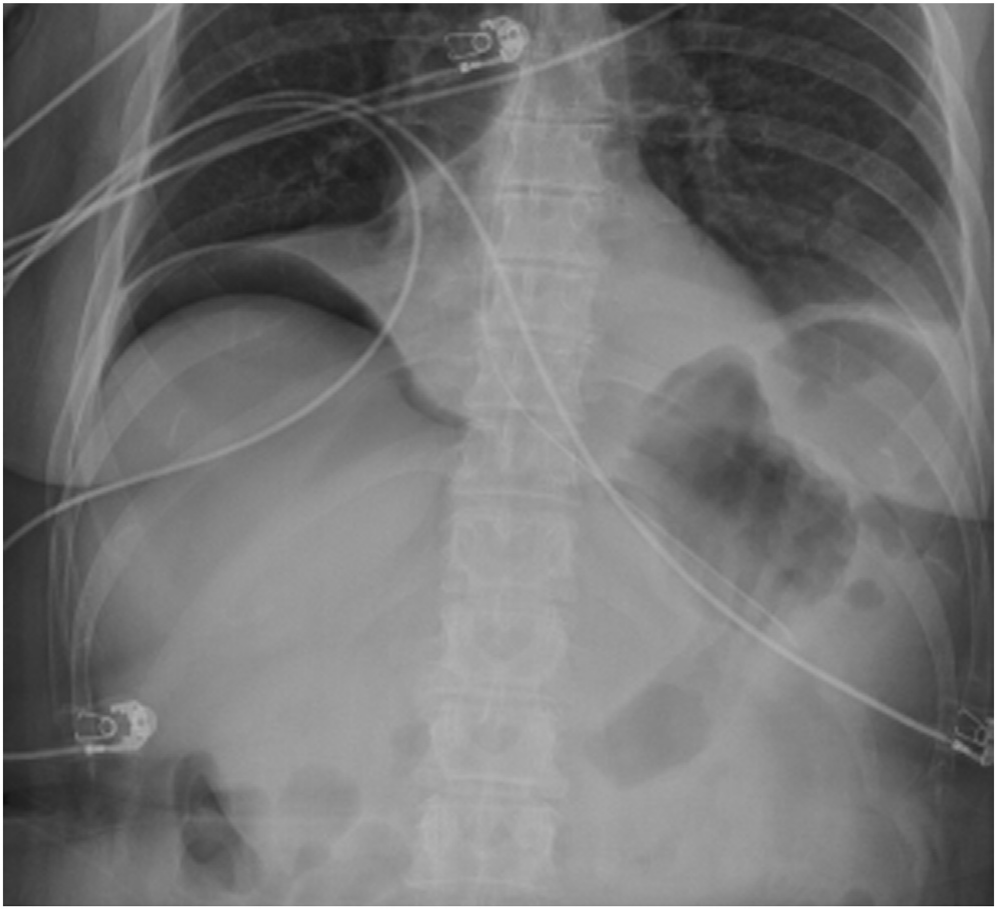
Abdominal X-ray portraying pneumoperitoneum after EGD.
